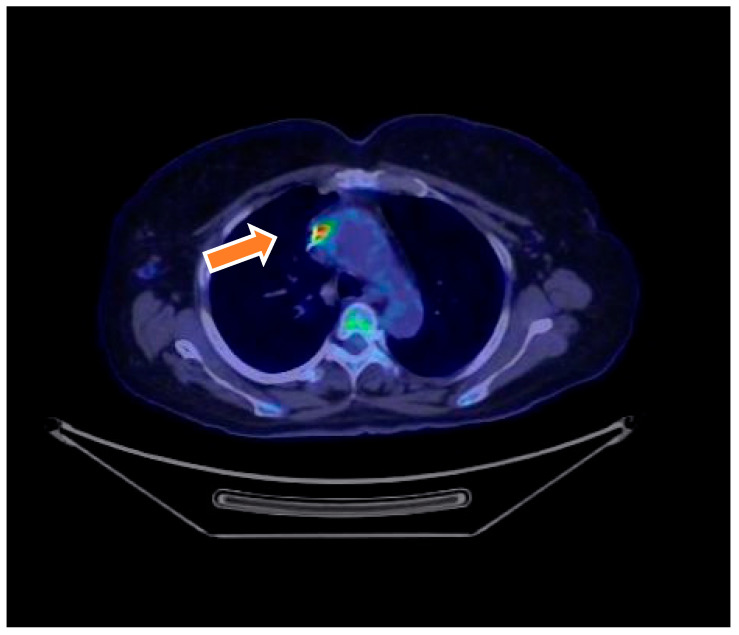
Transverse ^18^F-FDG PET/CT images show an infected prosthetic aortic valve. Culture after valve removal was positive for *Candida albicans*.
